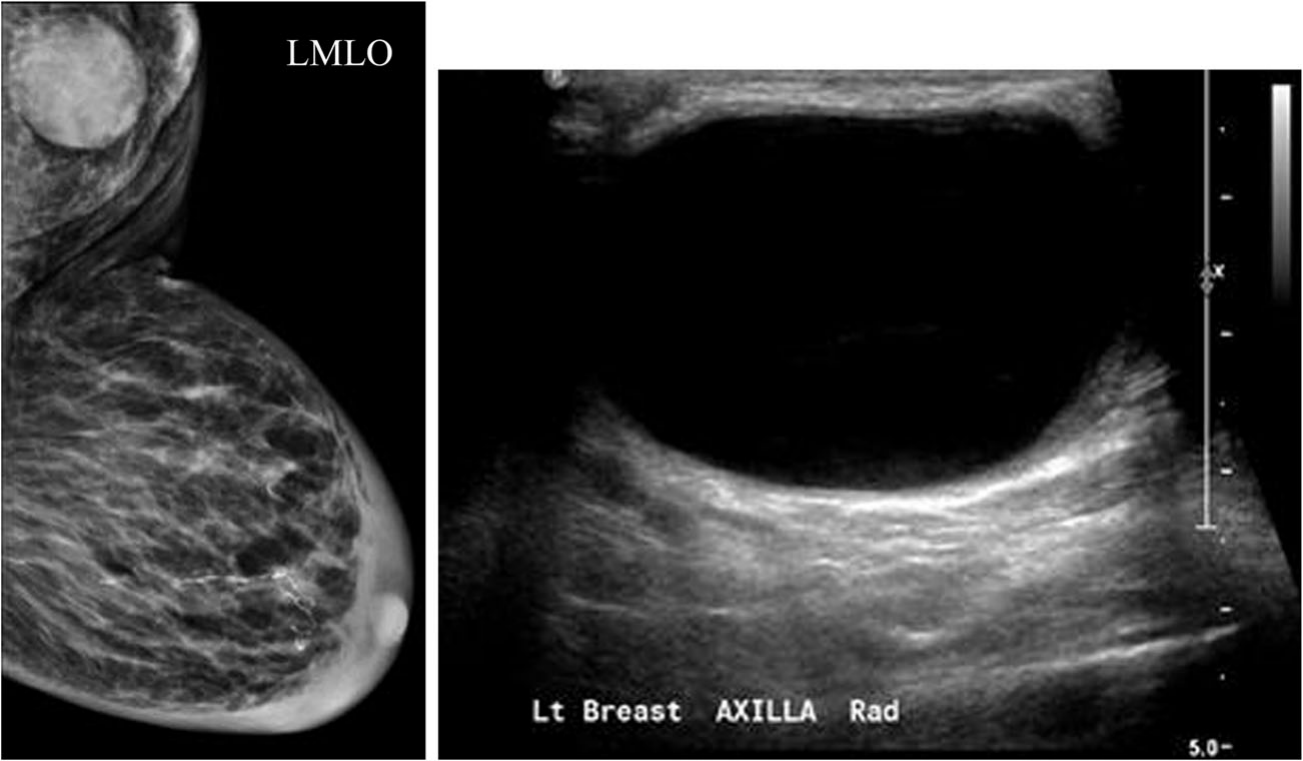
A 80-year-old female with a history of sarcoidosis presenting with a left axillary mass 1 year following axillary surgery of a benign node. a MLO view and (b) ultrasound of the left axilla demonstrates a 4.1 × 2.7 × 3.5-cm well-circumscribed predominantly anechoic structure with internal debris consistent with a complicated cyst. Aspiration was performed for symptomatic relief. Final diagnosis: lymphocele of the left breast demonstrates extensive skin and trabecular thickening and a well-circumscribed left axillary mass
